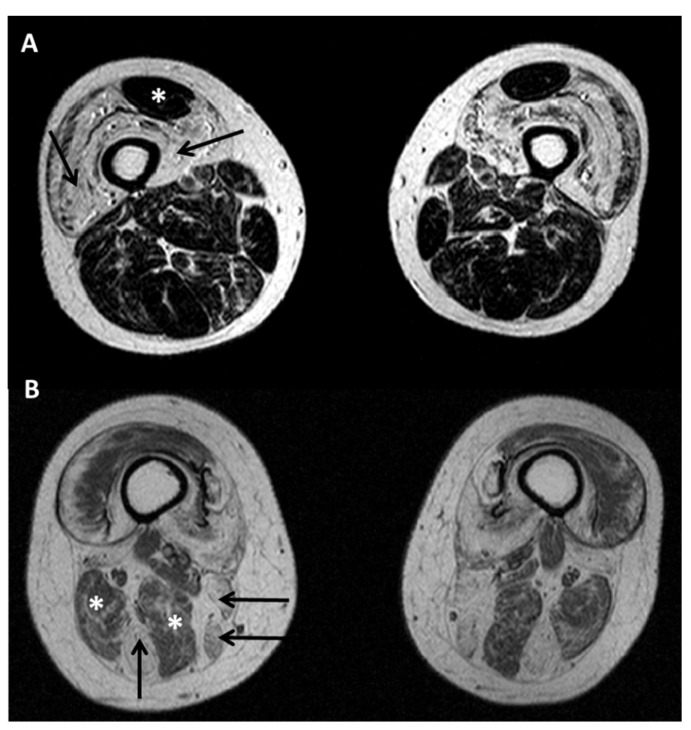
T1 weighted muscle MRI at thigh level in *LMNA* (A) and *DES* (B) mutated patients. (A) Marked fatty infiltration of the vasti (arrows) with sparing of the rectus femoris (asterisk) and of posterior compartment. (B) Marked fatty changes in semitendinosus, sartorius and gracilis (arrows) with relative sparing of long head of biceps femoris and semimembranosus (asterisks).
